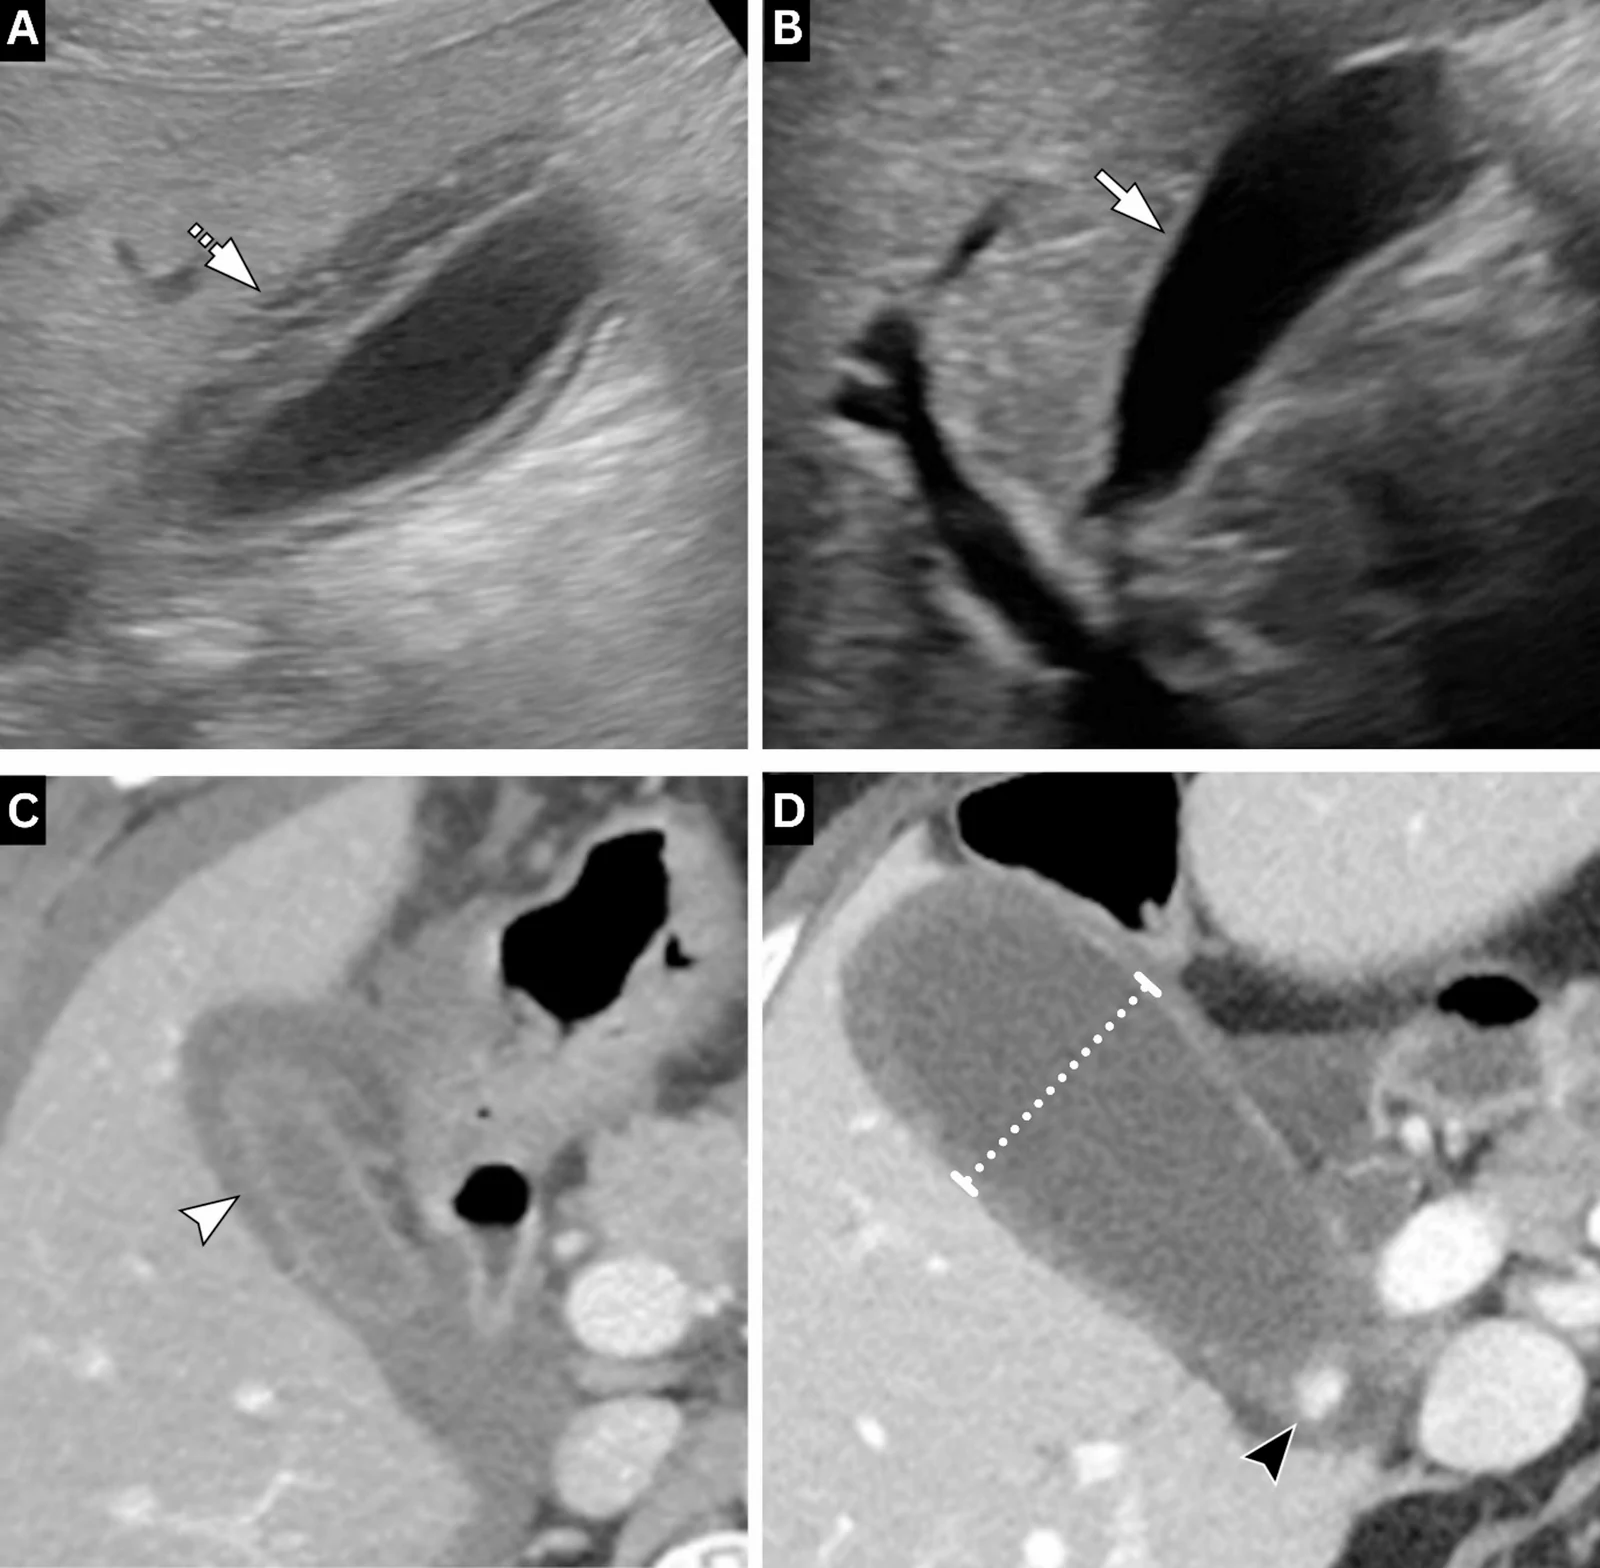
Gallbladder involvement in scrub typhus. A, B Transabdominal ultrasound shows diffuse gallbladder wall thickening with mural edema measuring up to 7 mm (white dotted arrow, A) but without tense luminal distension in a 29-year-old female, with near-complete resolution on the 3-week follow-up study (solid white arrow, B), consistent with treatment response. C, D Axial contrast-enhanced CT highlights the imaging distinction between scrub typhus-related gallbladder wall thickening and edema (white arrowhead, C) versus the tense luminal distension (dotted line, D) secondary to an obstructing gallstone in the neck (black arrowhead, D), and wall edema (measuring 4 mm) in acute calculous cholecystitis
